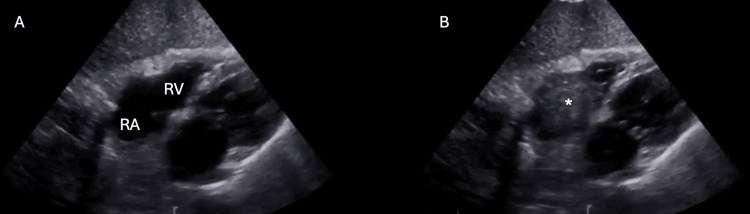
Subcostal echocardiography view with bubble test. A: Subcostal echocardiography view before the bubble test showing the Right Atrium (RA) and Right Ventricle (RV). B: Subcostal echocardiography view during the bubble test showing flow through the right heart (*).
